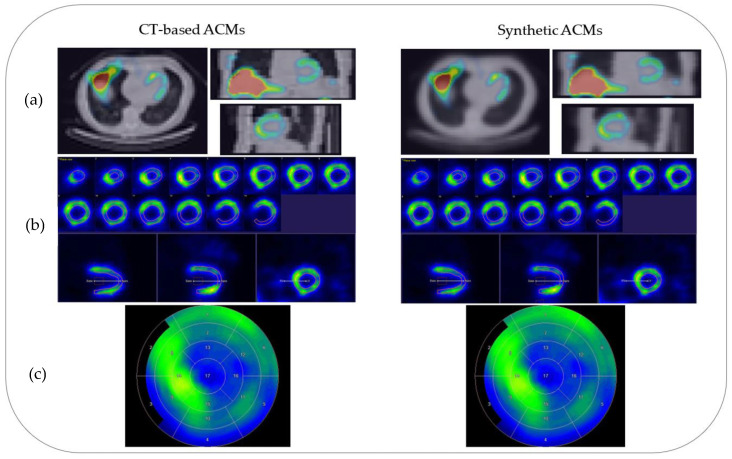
Sample results for a test patient. (a) AC-SC SPECT images fused with ACMs. (b) Short-axis slices. (c) Polar maps with 17 cardiac segments.
